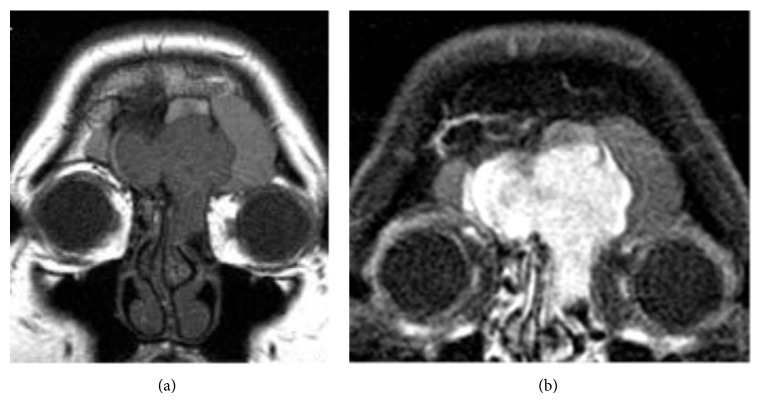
((a) and (b)) Preoperative coronal MRI images showing the tumor's frontal sinus location and (b) demonstrating tumor's strong contrast enhancement.
